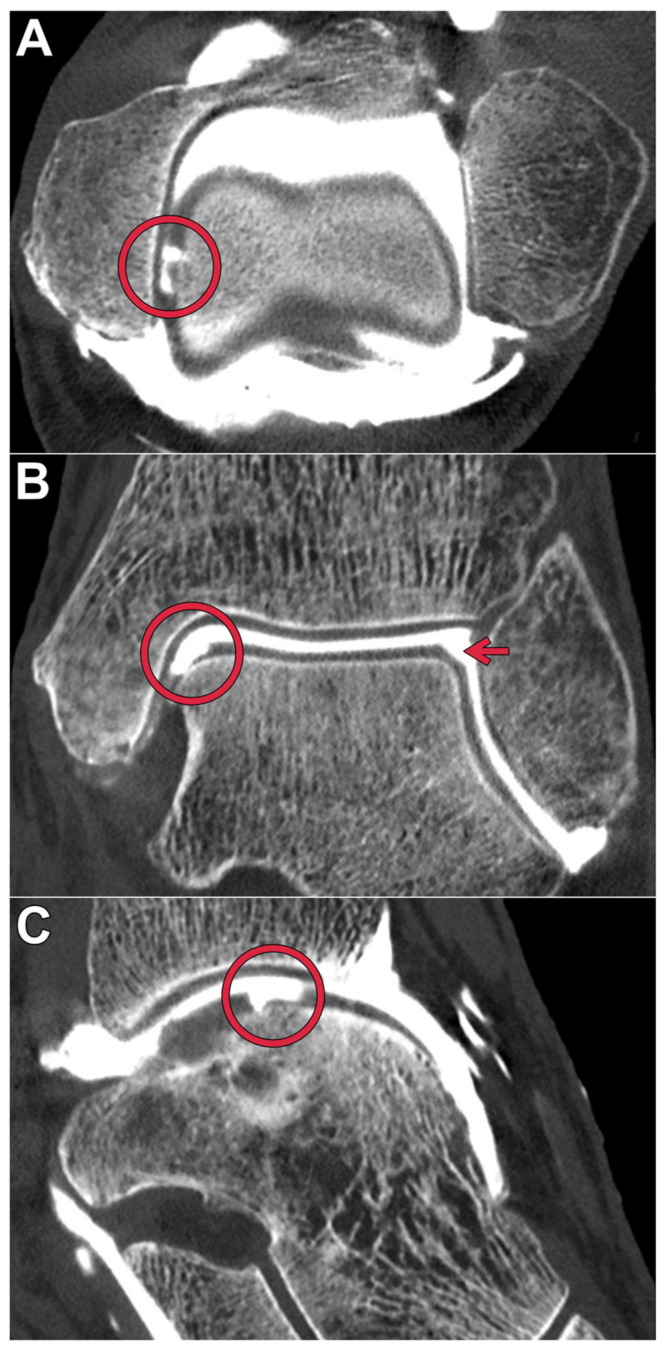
Tri-planar reformatting of a full-dose photon-counting CT arthrogram in axial (A), coronal (B), and sagittal orientation (C) using the medium BR84 kernel displays an osteochondral lesion of the medial talus shoulder (red circle). Additionally, a partial thickness cartilage injury of the lateral talus shoulder can be diagnosed (red arrow).
